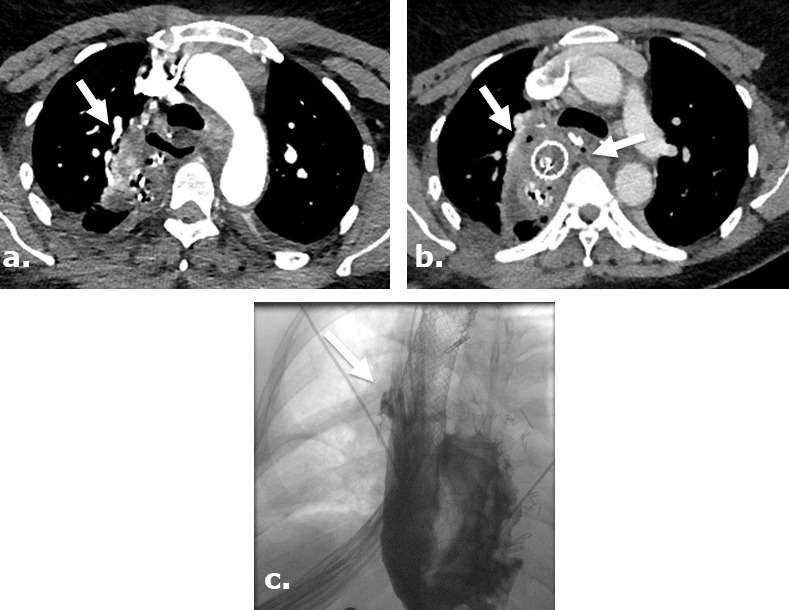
Anastomotic leak after Ivor Lewis esophagogastrectomy in a surgical case where Surgicel was not used. Axial images of a contrast-enhanced CT obtained 5 days after surgery (a) in a 46-year-old female with a history of stage I esophageal adenocarcinoma, demonstrates extra luminal gas anterior to the enteric tube. An axial view of contrast-enhanced CT obtained 9 days after surgery (b) demonstrates persistent gas and fluid surrounding the now stented gastric pull through. Single upper gastrointestinal series with water-soluble contrast obtained 13 days after surgery (c) demonstrates persistent leak at the level of esophagogastric anastomosis.
